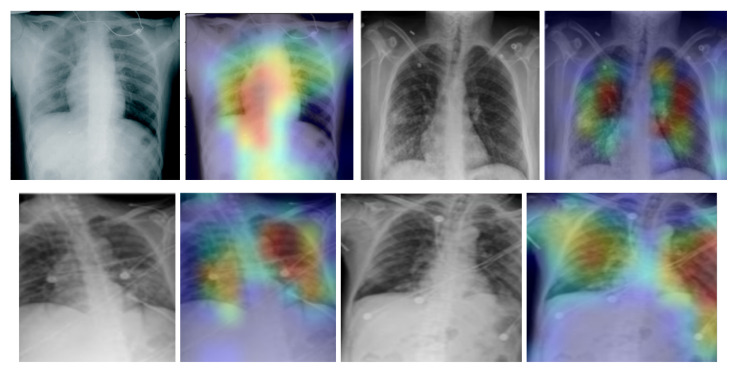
Grad-CAM visualisation for the COVID-19 class using the EfficientNetB4-based Approach 1 model for four chest X-ray images.
